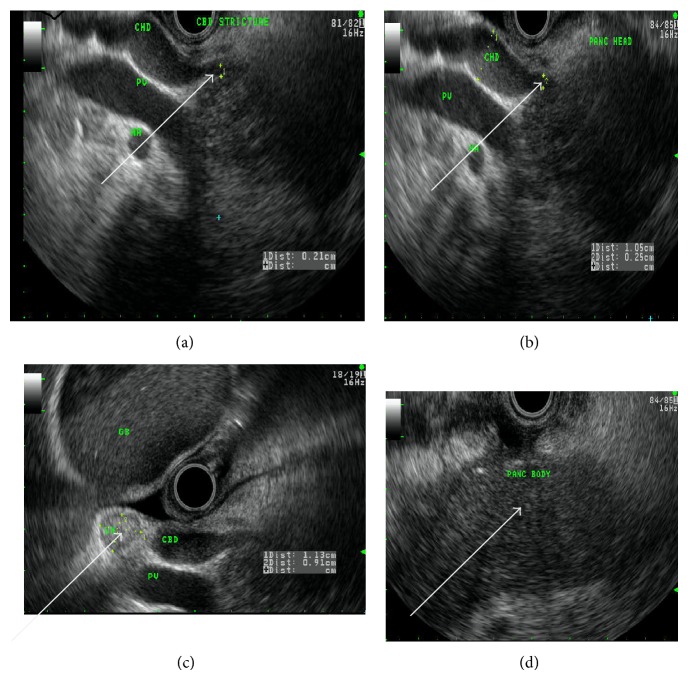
Endoscopic ultrasound images arrows showing (clockwise) (a) CBD stricture, (b) dilated CHD, (c) reactive lymph node, and (d) homogenous pancreatic body.
